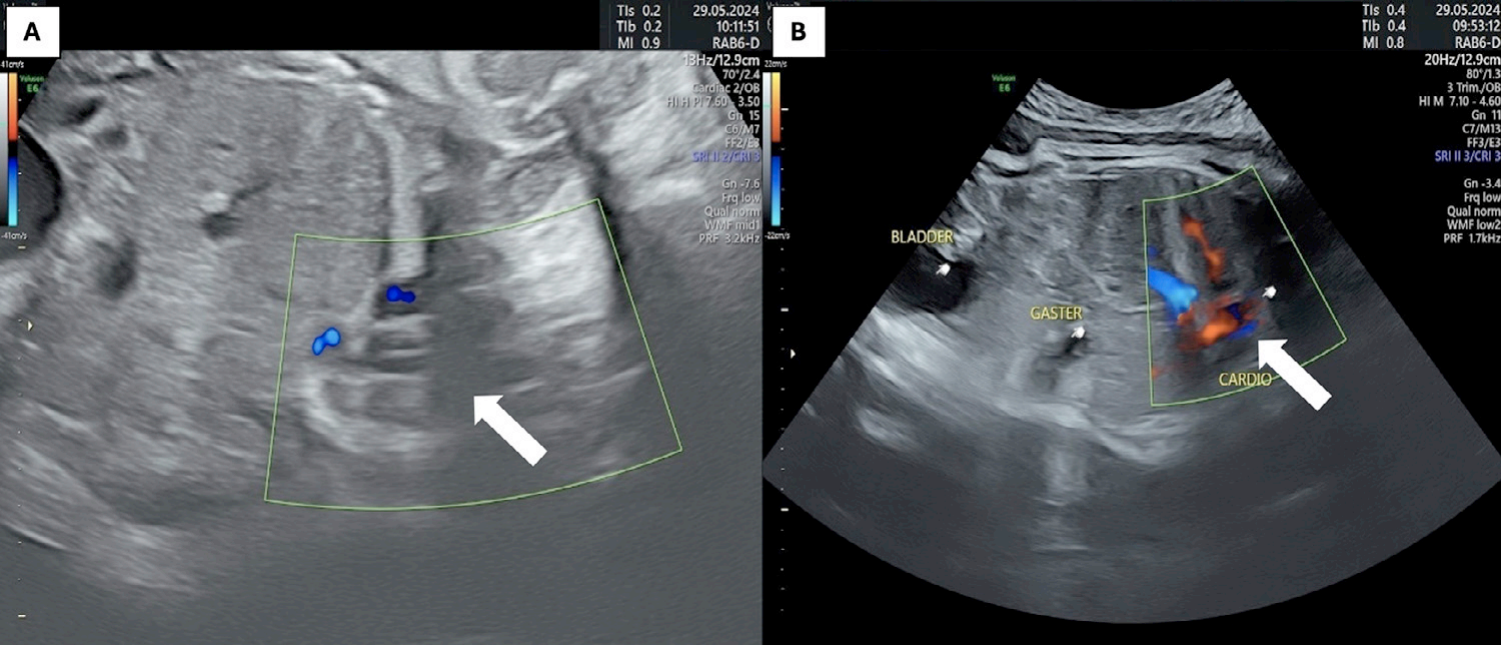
Ultrasound showing that atriums and ventricles lie outside the chest cavity the heart is located completely outside the chest cavity (white arrow), with a normal 4-chamber appearance and a regular heartbeat. In the abdomen, the stomach was normal, the diaphragm was intact, the kidneys and bladder were normal during sonography and normal umbilical cord insertion was noted.
